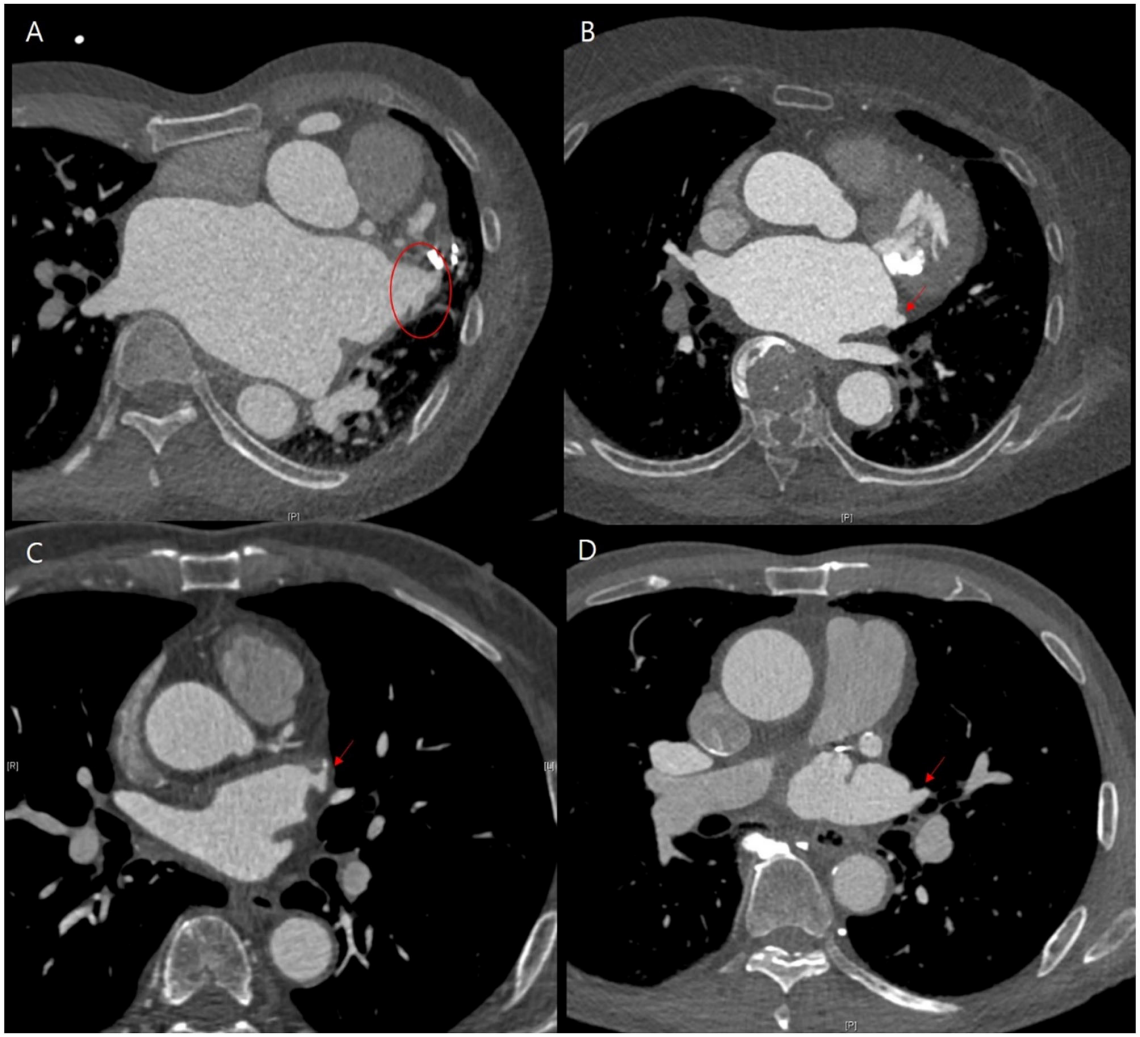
Representative cases of follow-up computed tomography (CT) findings that showed residual left atrial appendage (LAA). (A,B) Patients exhibited the occurrence of ischemic stroke, while (C,D) did not. (A) Residual trabeculated LAA. (B) Left atrial accessory appendage and mitral annular calcification without functional mitral stenosis. (C,D) Remnant LAA pouch.
